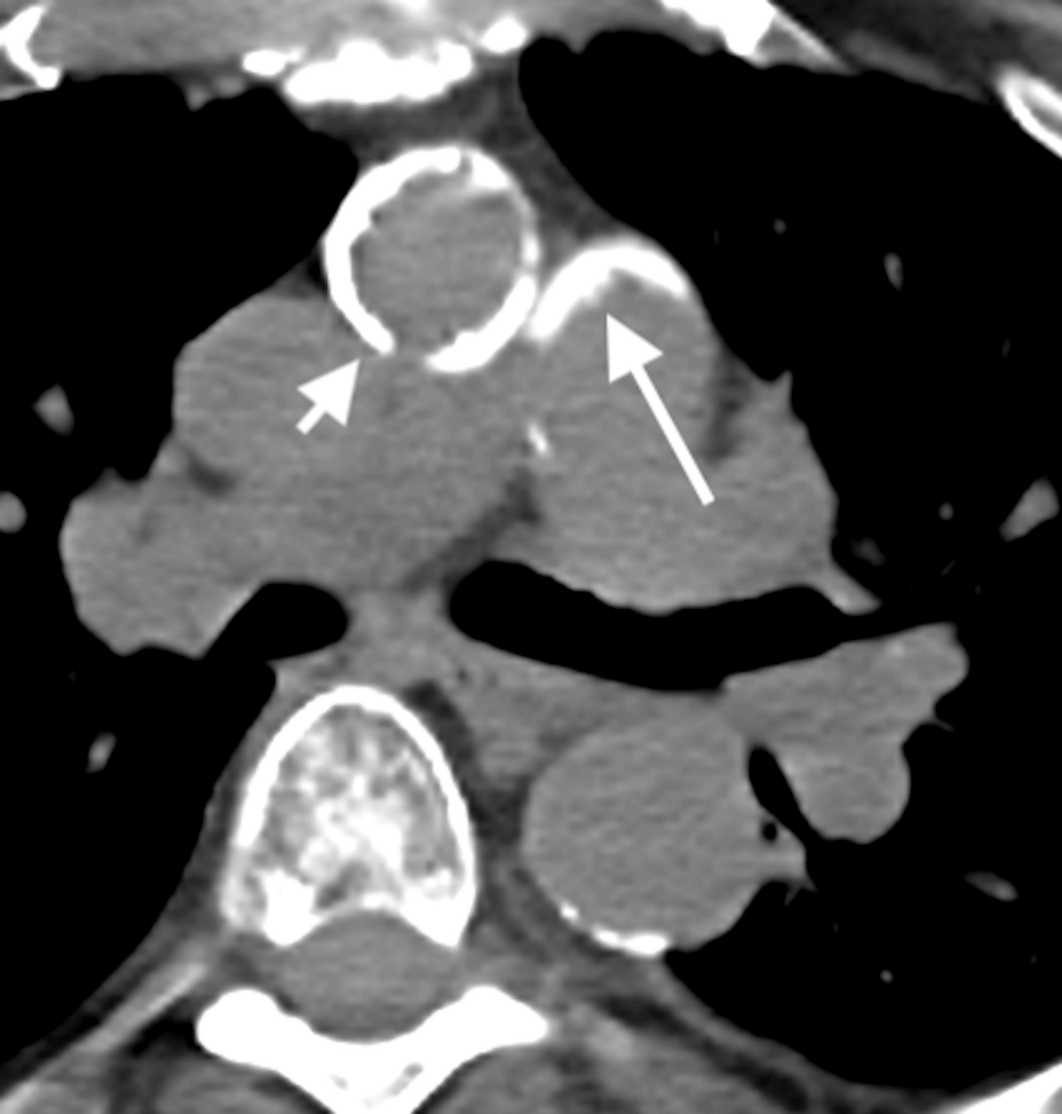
Axial non-contrast CT in soft tissue window through the mediastinum. Shows severe atherosclerotic calcifications of the ascending aorta (short arrow) and severe calcification of the pulmonary trunk (long arrow) with mild extension into the left main pulmonary artery.
